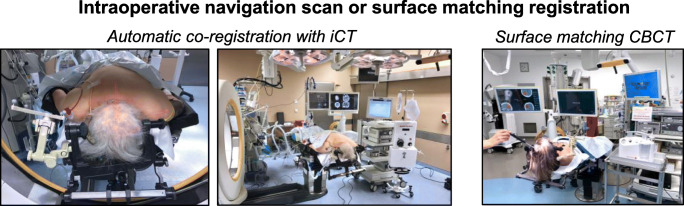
Positioning and navigation registration. The left panels show the iCT setup for automatic patient/image co-registration in a case without preoperative registration CT (patient 1): For iCT scanning, the head is fixed in a radiolucent clamp that holds the navigation tracking device and the patient is rotated into the iCT gantry (left). For surgery, the OR table is rotated into surgery position (center). The right panel shows the CBCT setup for surface matching registration in a case with preoperative registration CT (patient 15)
